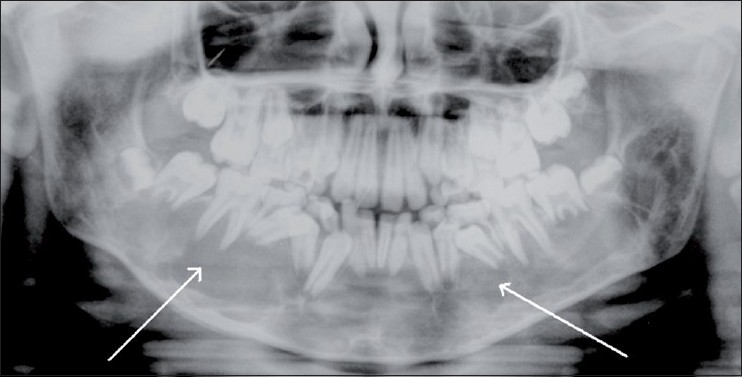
Orthopantomogram shows a large expansile osteolytic lesion (arrows) involving both sides of the body of the mandible
